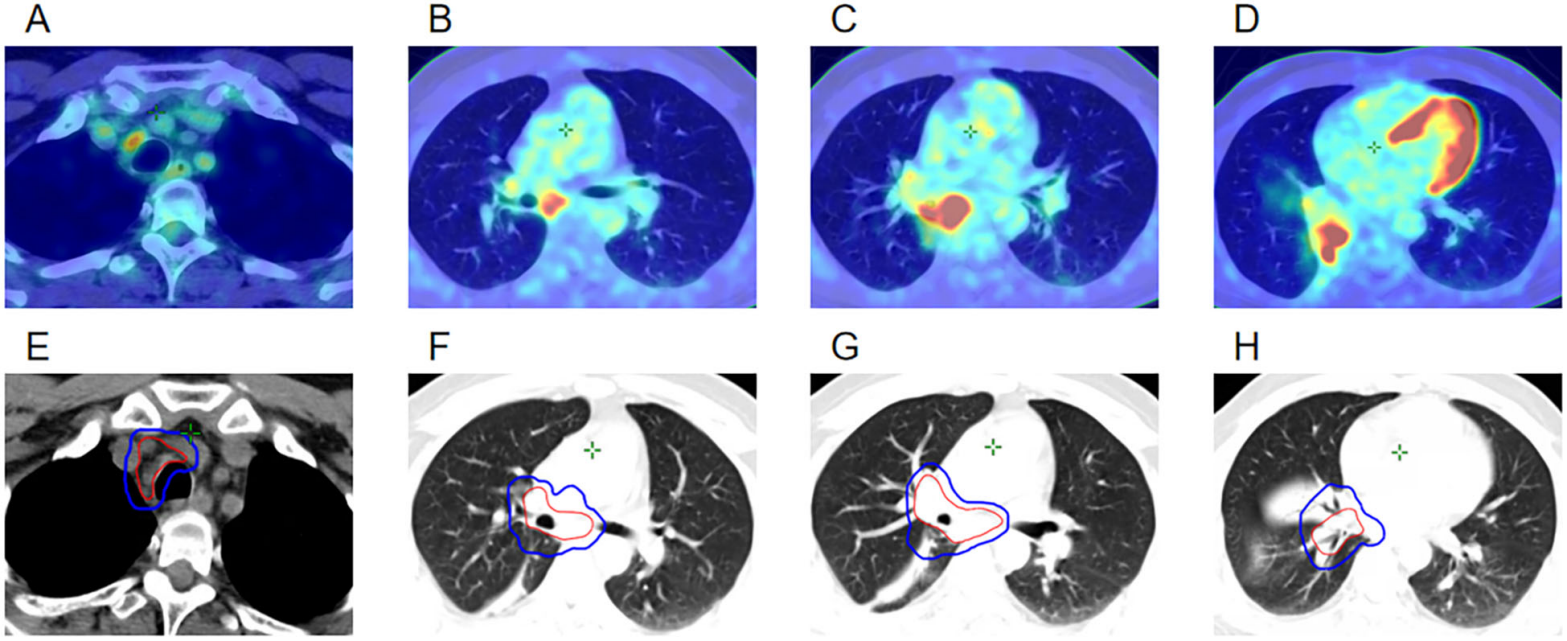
(A–D) PET-CT images at the time of initial diagnosis in March 2023. (E–H) Clinical target volume and planning target volume of the radiotherapy target area in April 2023.
